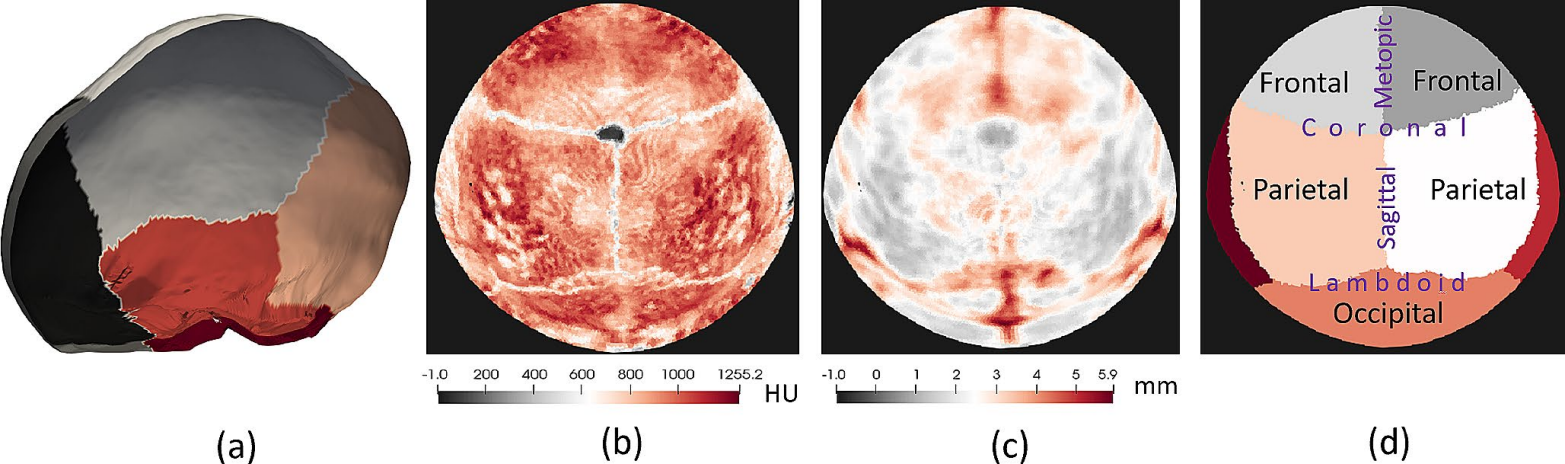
Standardized representation of the calvaria. (a) Cranial surface segmented from a CT image with color-coded bone labels. (b)-(c) Standardized 2-dimensional representation of the average bone density in Hounsfield units (b) and thickness in mm (c) for a 1.4-year-old normative subject. (d) Location of every major bone and suture
